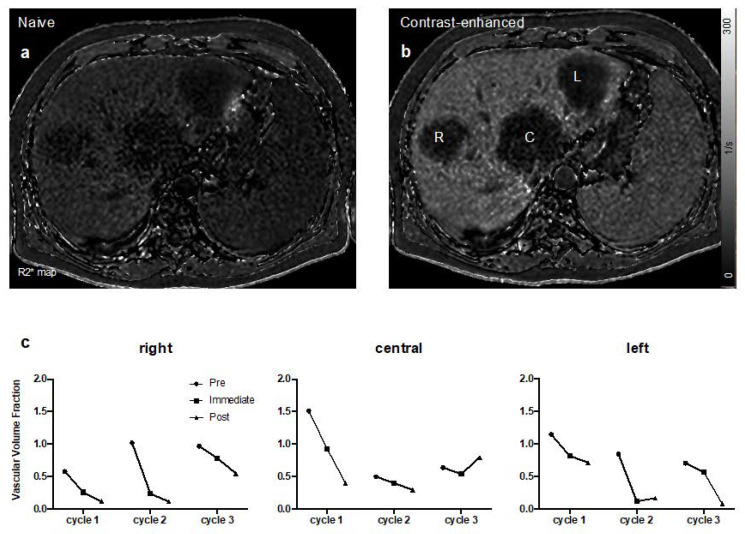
Early evaluation of tumor perfusion response to tTF-NGR using R2* mapping in MRI: R2* maps before (a) and after (b) injection of the iron-based contrast medium Resovist^®^ visualize the perfusion of 3 liver metastases (R = right, C = central, and L = left) of a patient with a colorectal carcinoma (UPN 007). The calculated vascular volume fractions (VVF) demonstrate a reduction in blood flow after treatment with tTF-NGR for each of the 3 cycles (c) at 3 (cycle 1), 4 (cycle 2), and 5 (cycle 3) mg/m^2^ tTF-NGR per day × 5. Notice the partial reperfusion at the beginning of cycles 2 and 3. (c) y-axis shows arbitrary units, black circles represent measurements before (pre) treatment with tTF-NGR, black squares are 5 h (immediate) after tTF-NGR, and black triangles are after 5 days (post) of tTF-NGR at the end of a cycle.
